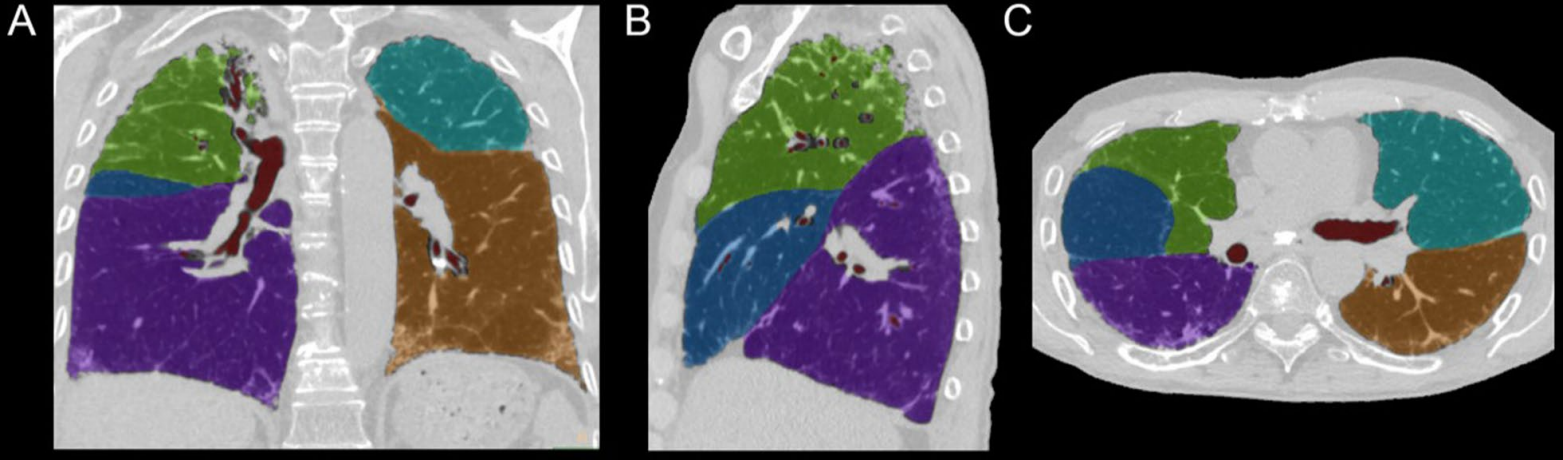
Measurement of lobar lung volume using COPD software (IntelliSpace Portal; Philips Healthcare) of chest HR computed tomography (DECT) images (A: coronal, B: Sagittal, C: axial). A specific color has been attributed for each lobe, and lobar volume was derived
